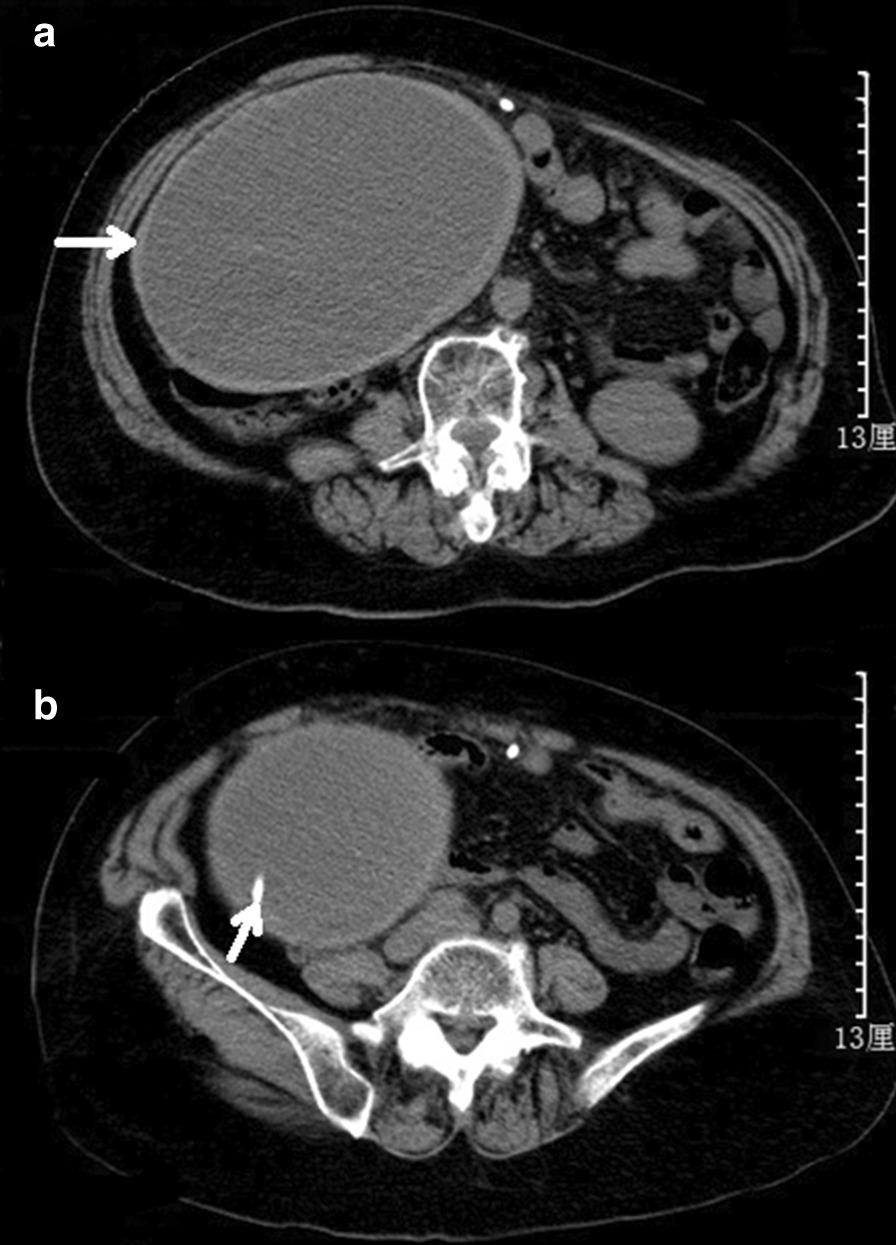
a Abdominal CT demonstrated a large cyst comprising a collection of homogeneous iso-density fluid in the abdominal cavity with displacement of the bowel loops to the posterior part. b The distal segment of the ventriculoperitoneal shunt catheter was located within the cystic mass with clear margins. The cyst and the shunt catheter were indicated by white arrows
